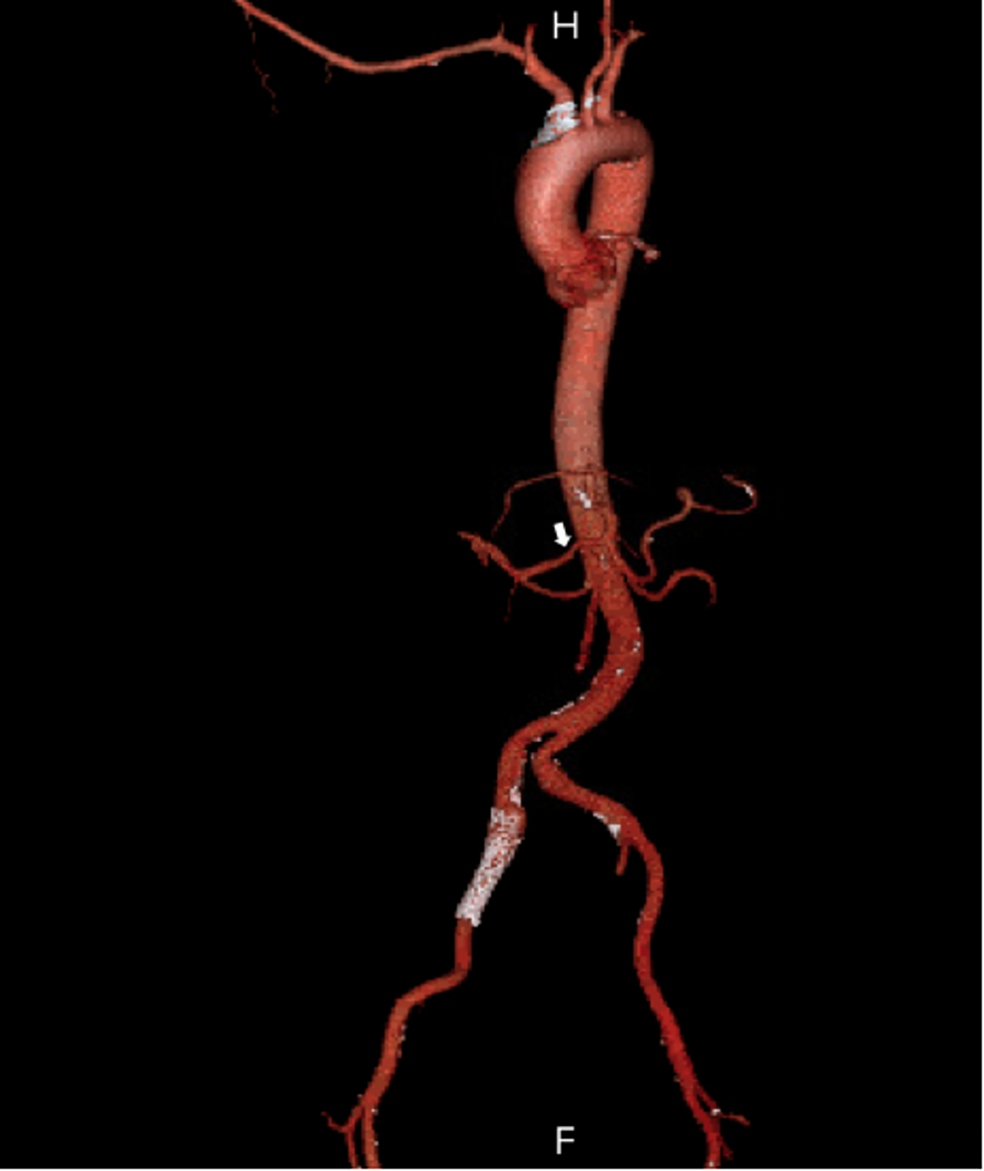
A CT angiogram demonstrates a mildly narrowed celiac axis with a near-completely replaced hepatic artery off of the superior mesenteric artery (SMA) and an accessory left hepatic artery off of the left gastric artery (normal variant).
